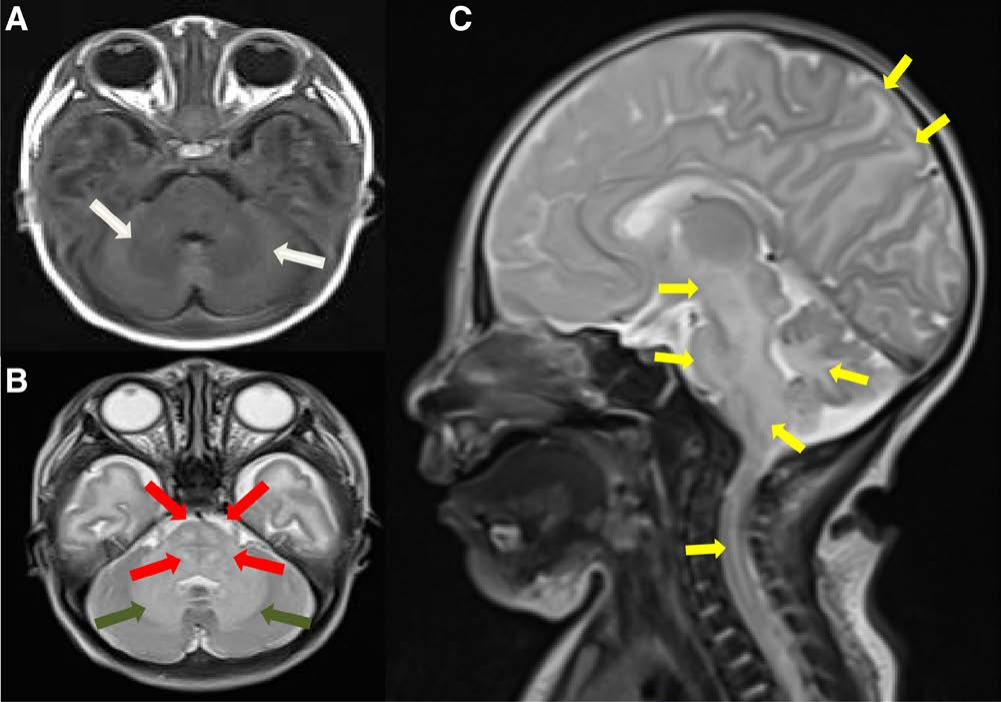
The brain T1WI and T2WI images of an 8-day-old female with MSUD. (a) The cerebellum and pons showed slightly symmetric low signal intensity (white arrow) on T1WI of cranial transection. (b) The corresponding parts of T2WI showed slightly high signal intensity (cyan arrow), and the pons showed symmetrical circular high signal intensity (red arrow), which looked like “button”. (c) The median sagittal MRI of the brain showed there were extensive patches and cords of T2 hyperintensity in the anterior and posterior central gyrus, cerebellar dentate nucleus, brainstem, and cervical spinal cord (yellow arrow). MSUD = maple syrup urine disease, MRI = magnetic resonance imaging, T1WI = T1 weighted imaging, T2WI = T2 weighted imaging.
